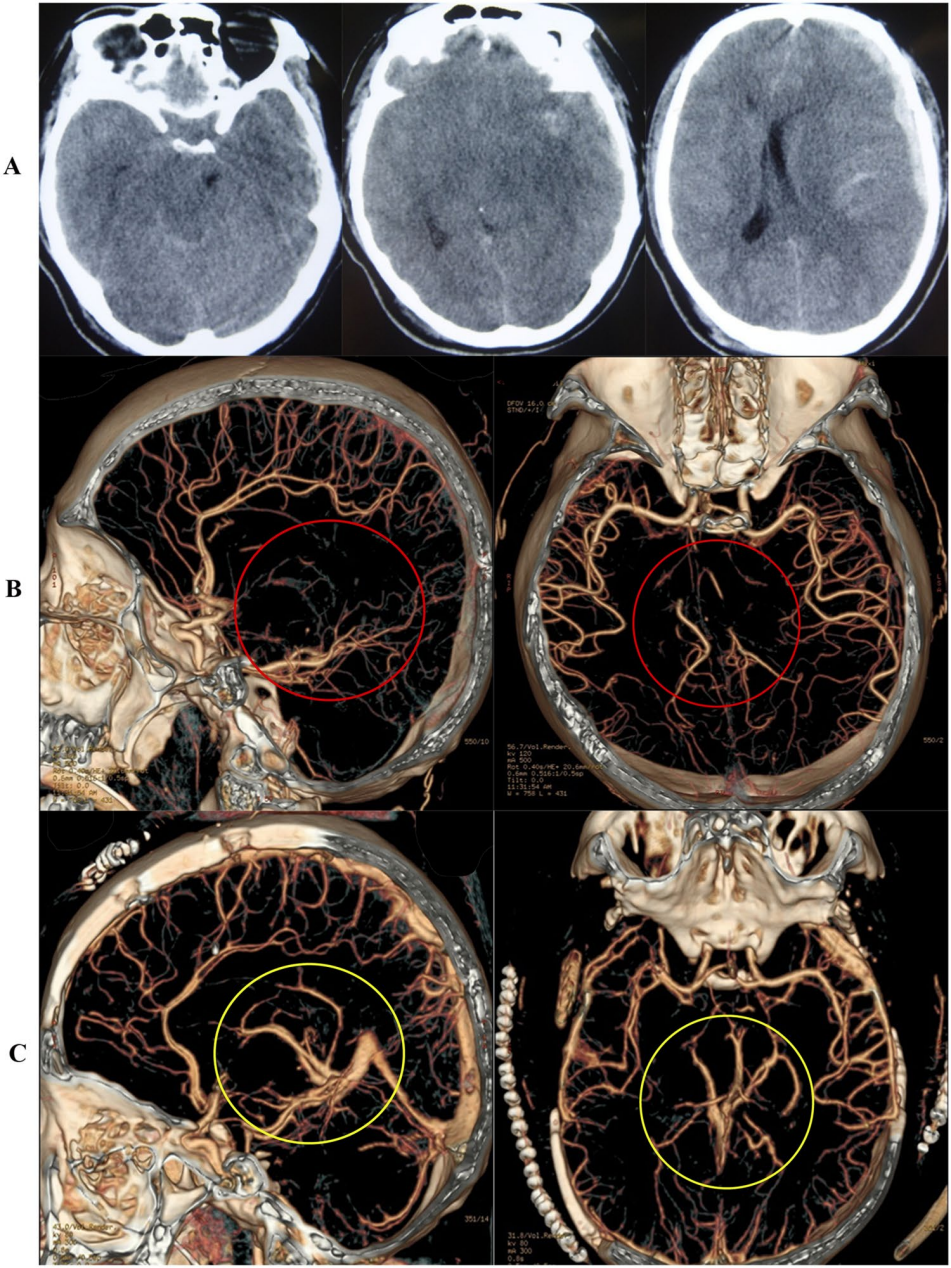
DVSO of CTA. A case of a severe TBI patient with GCS 4. (A) CT indicates subdural hematoma and diffuse brain swelling. (B) Preoperative CTA indicates intracranial deep venous system occlusion (red circle). (C) Postoperative CTA indicates intracranial deep venous system improvement (yellow circle).
